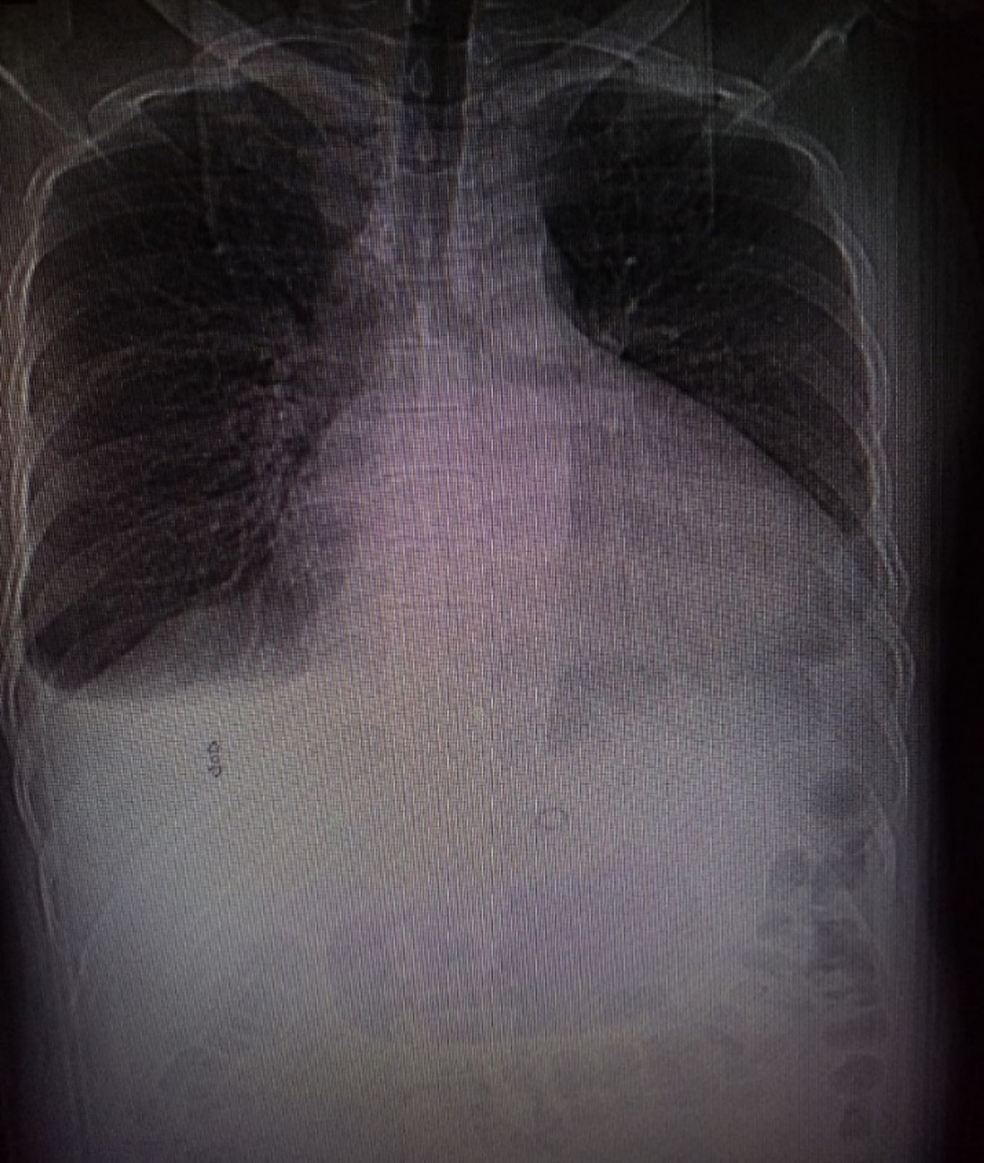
X-ray chest on day six of admission to ICU: showing minimal right-sided pleural effusion
